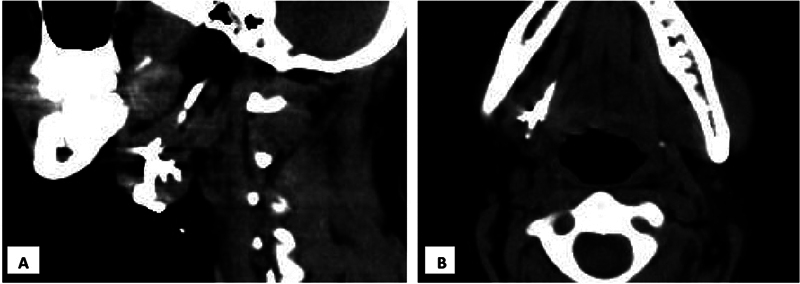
Important computed tomography fistulography findings. (A) Sagittal view: a vertical tract extending from the neck skin to the intraglandular parenchyma corresponding with sialo-cutaneous fistula, and a horizontal tract converging from the intraglandular region that represents the Wharton duct. (B) Axial view: Horizontal tract extending from the submandibular gland till the floor of the mouth, consistent with Wharton duct.
